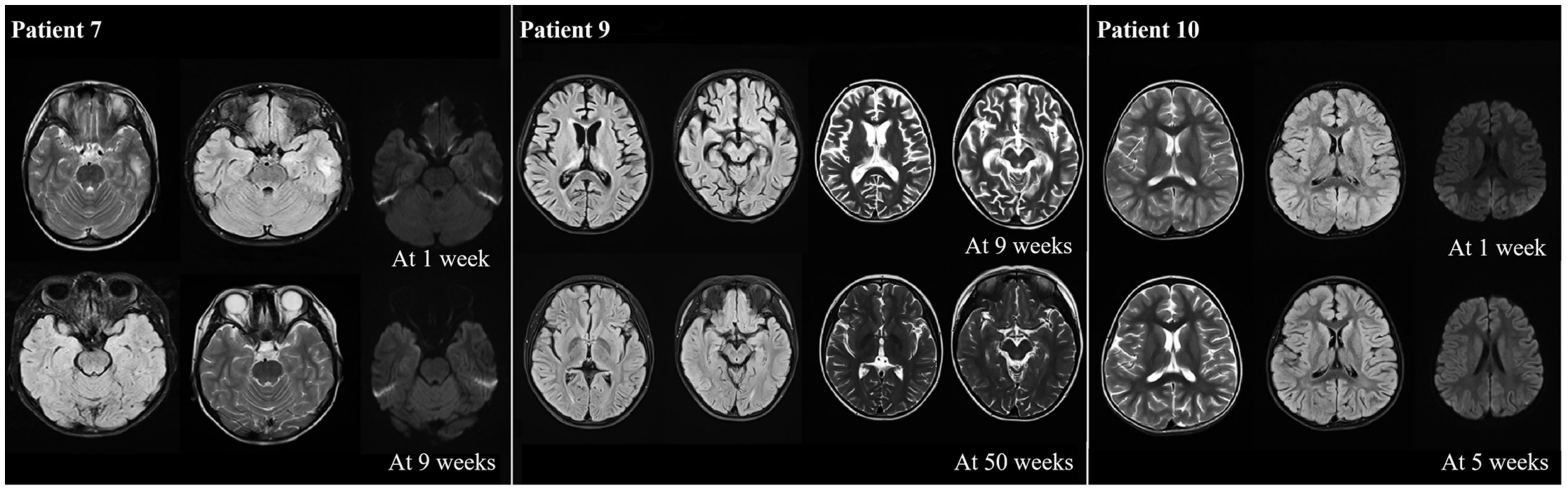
Abnormal neuroimaging findings in patients with anti-CASPR2 Ab-related encephalitis. Patient 7 with abnormal signalin bilateral frontal left temporal cortex and subcortical at one week after disease onset. Compared with onset, the left temporal cortex and subcortical signals were significantly reduced at 9 weeks after onset; the neuroimaging of P9 revealed bilateral thalamus, brain foot small sheet of abnormal signal, brain groove fissure slightly wide and deep, bilateral lateral ventricle slightly enlarged at 9 weeks from oneset, all symptoms improved at 50 weeks from oneset; the neuroimaging of P10 displayed bilateral caudate head, multiple T2 abnormalities in bilateral frontal, temporal and occipital cortex, FLAIR hyperintensity, and limited diffusion at one week after onset. After 5 weeks of treatment, all lesions decreased compared to one week of onset. CASPR2, Contactin-Associated Protein-like 2; Ab, antibody; MRI, Magnetic Resonance Imaging; FLAIR, fluid attenuated inversion recovery.
